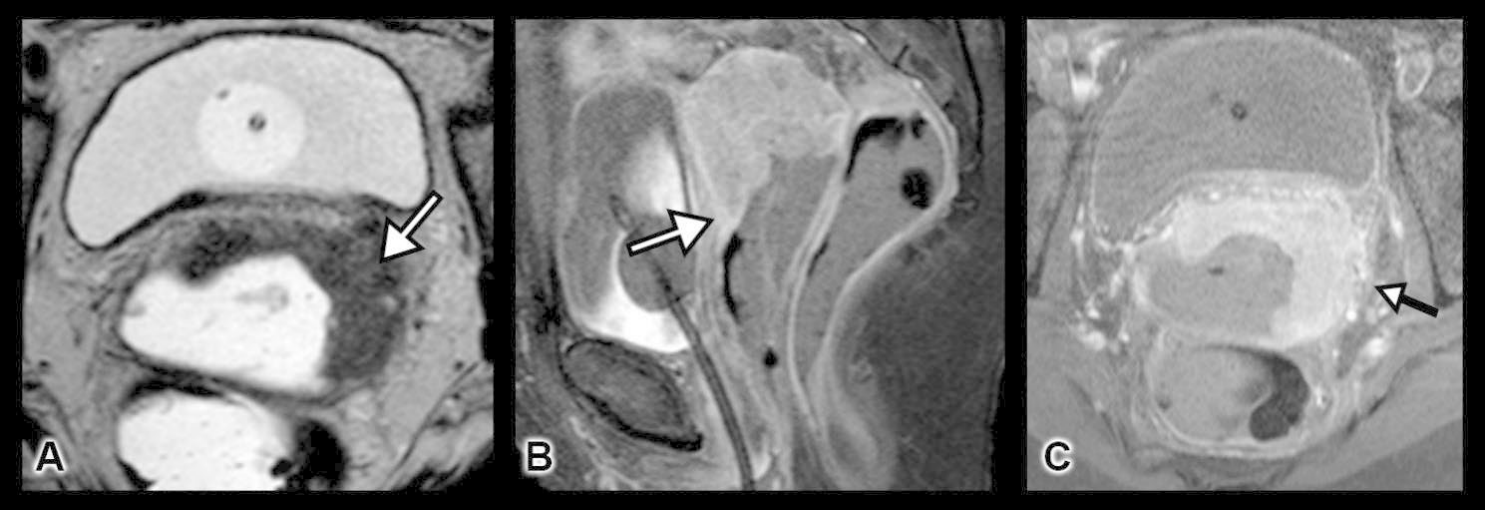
A) Axial T2-weighted MRI shows a large cervical mass with enrcirclement of the left ureter and subtle mucosal edema of the urinary bladder. Vaginal and rectal gel instilled prior to examination. Foley catheter in situ. B) Sagittal contrast-enhanced T1-weighted MRI with fat saturation shows a homogenously enhanced cervical mass expanding into the vaginal vault. C) Axial oblique contrast-enhanced T1-weighted MRI with fat saturation shows the circular tumor growth of the cervical mass. In this patient a bilateral parametrial invasion is present (Right side not shown)
